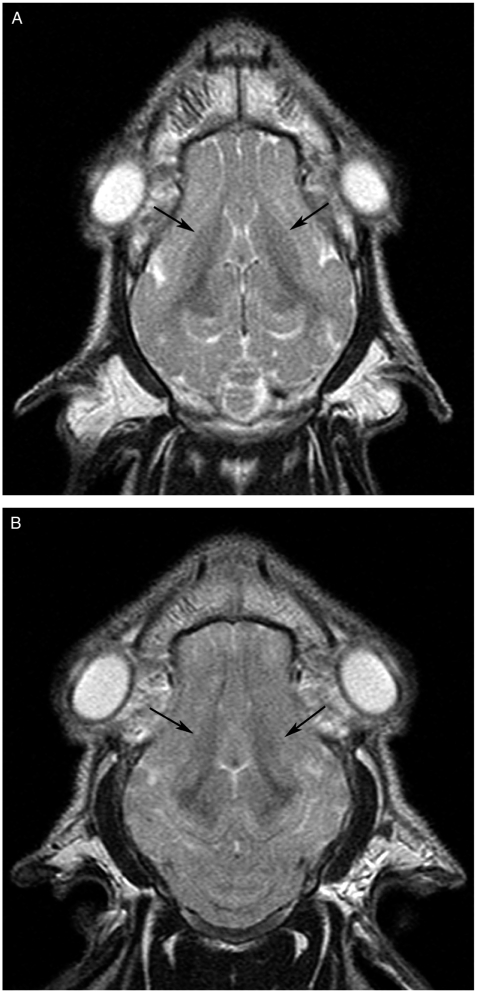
Morphological changes visualized by T2 in the basal ganglia. Axial T2-weighted spin-echo images (TR/TE 4000/90 ms) of subject 8 prior to HI (A) and 7 hours after HI (B). The most prominent morphological change seen on the MR images after 7 hours was a slight relative increase in signal intensity in the basal ganglia with effacement of borders post-HI (arrows).
